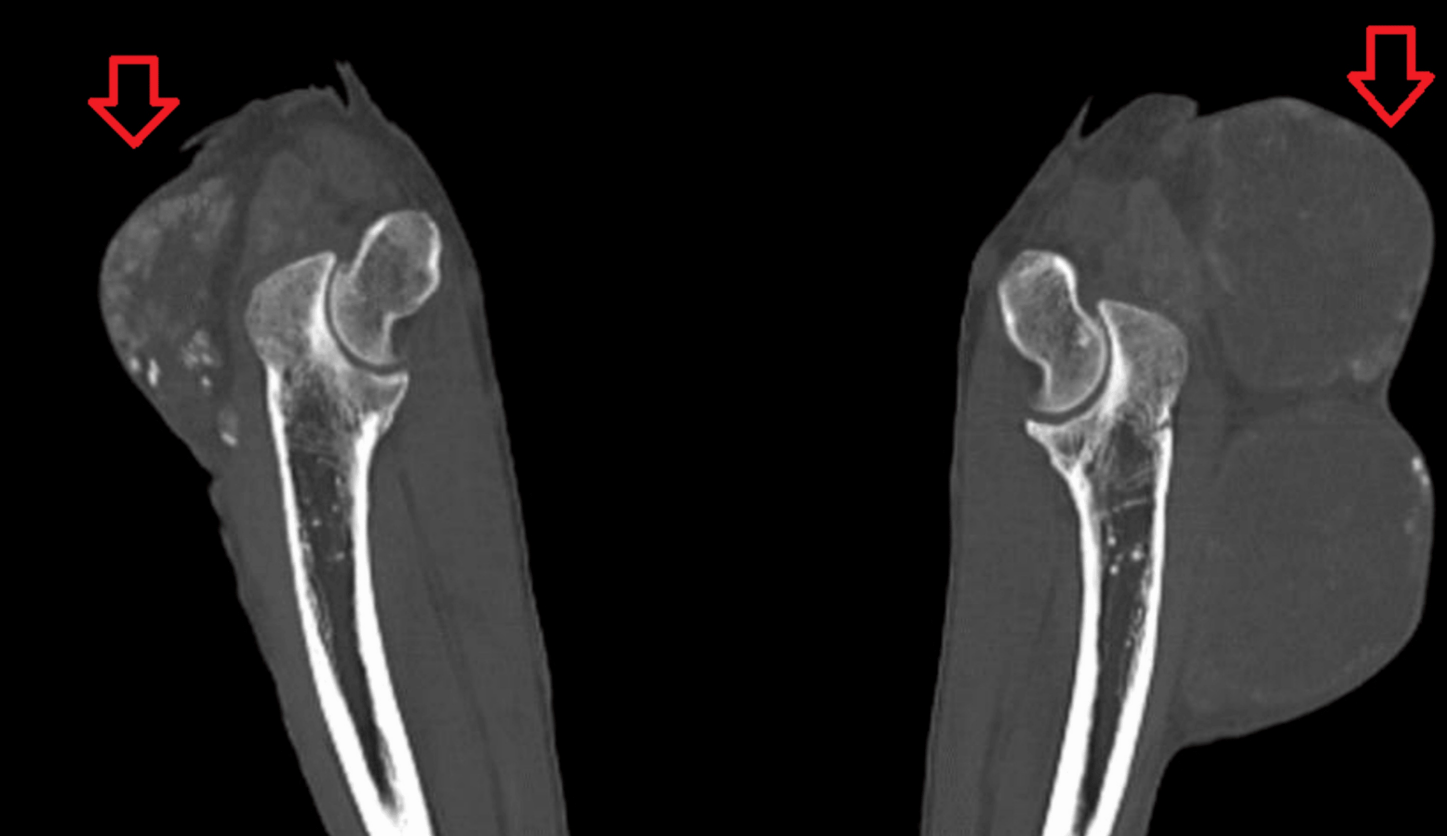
CT scan sagittal view of both elbows showing bilateral soft tissue fluid-filled cystic masses
